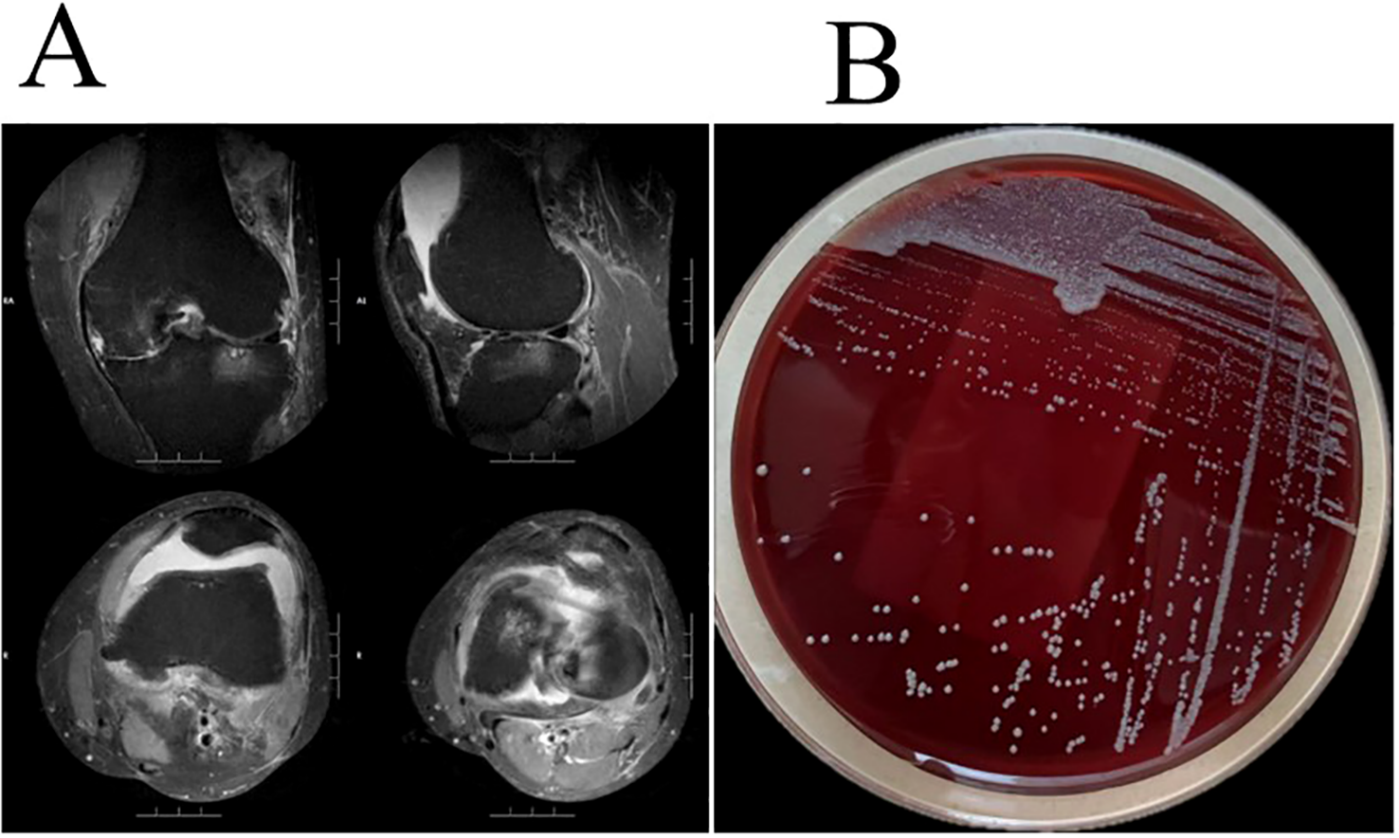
(A) Left knee MRI revealed osteoarthritis, a medial meniscus tear, degeneration of the lateral meniscus, and joint effusion. (B)
